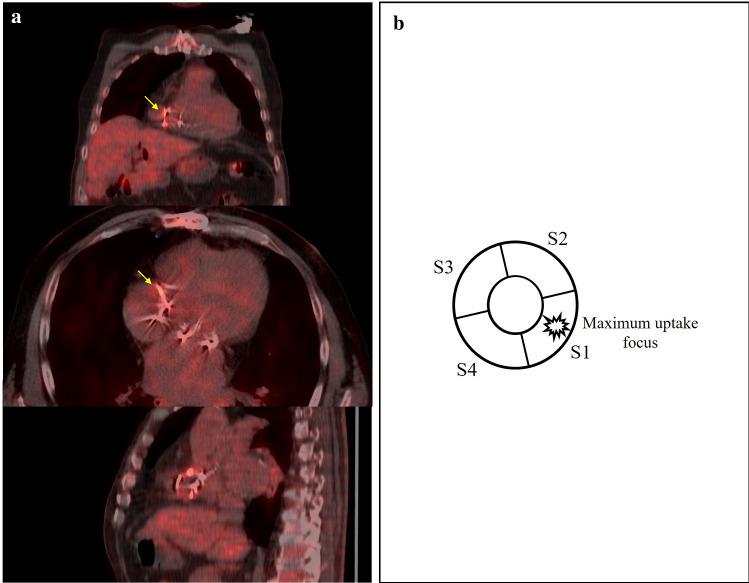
a [^18^F]FDG PET/CT image in coronal, axial and sagittal slices showing focal enhancement over the aortic prosthetic valve, difficult to objectify and localize in the visual analysis. b Prosthetic ring correct orientation and definition of the 90° segments. Counterclockwise definition of the 90° segments regarding to the maximum uptake focus, allocated in the center of the first segment
